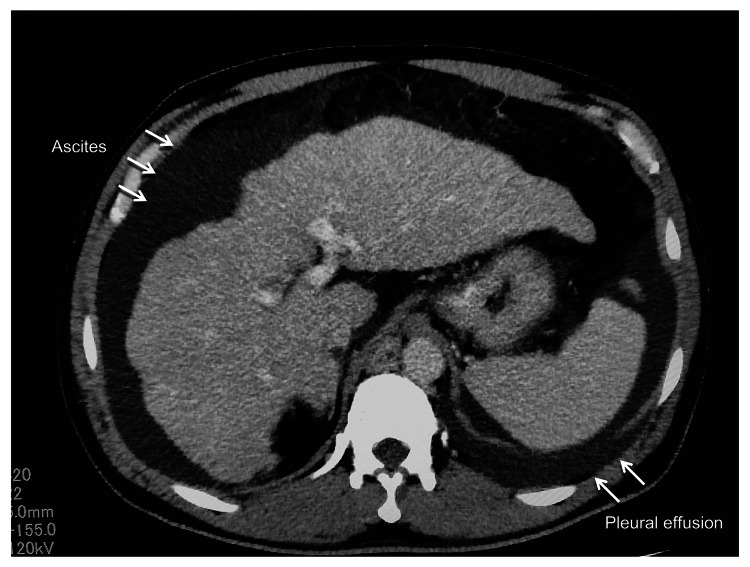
CT imaging findings CT, computed tomography
